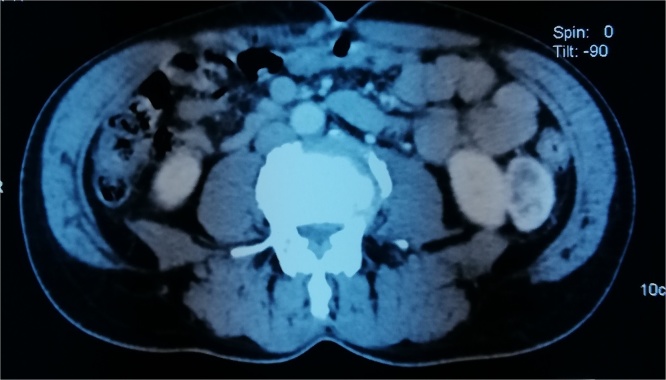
CT revealing an exophytic lesion in the lower pole of the left kidney, measuring 36 × 23 × 39 mm, well demarked, with peripheral enhancement, and a central fluid collection.
